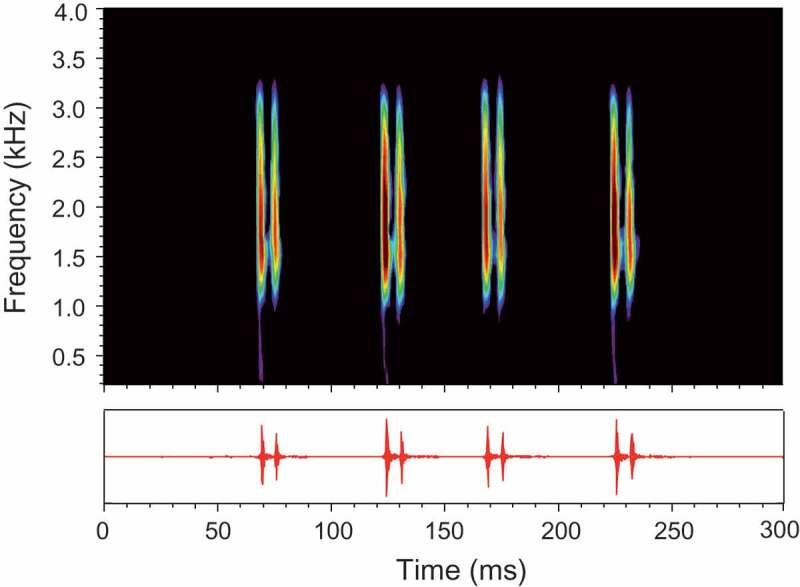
Sonogram (above) and oscillogram (below) of an agonistic sound emitted by a female *T. schalleri*. The sound consists of four double-pulsed bursts. Sampling frequency 16 kHz, filter bandwidth 180 Hz, 75% overlap, Hanning window.
